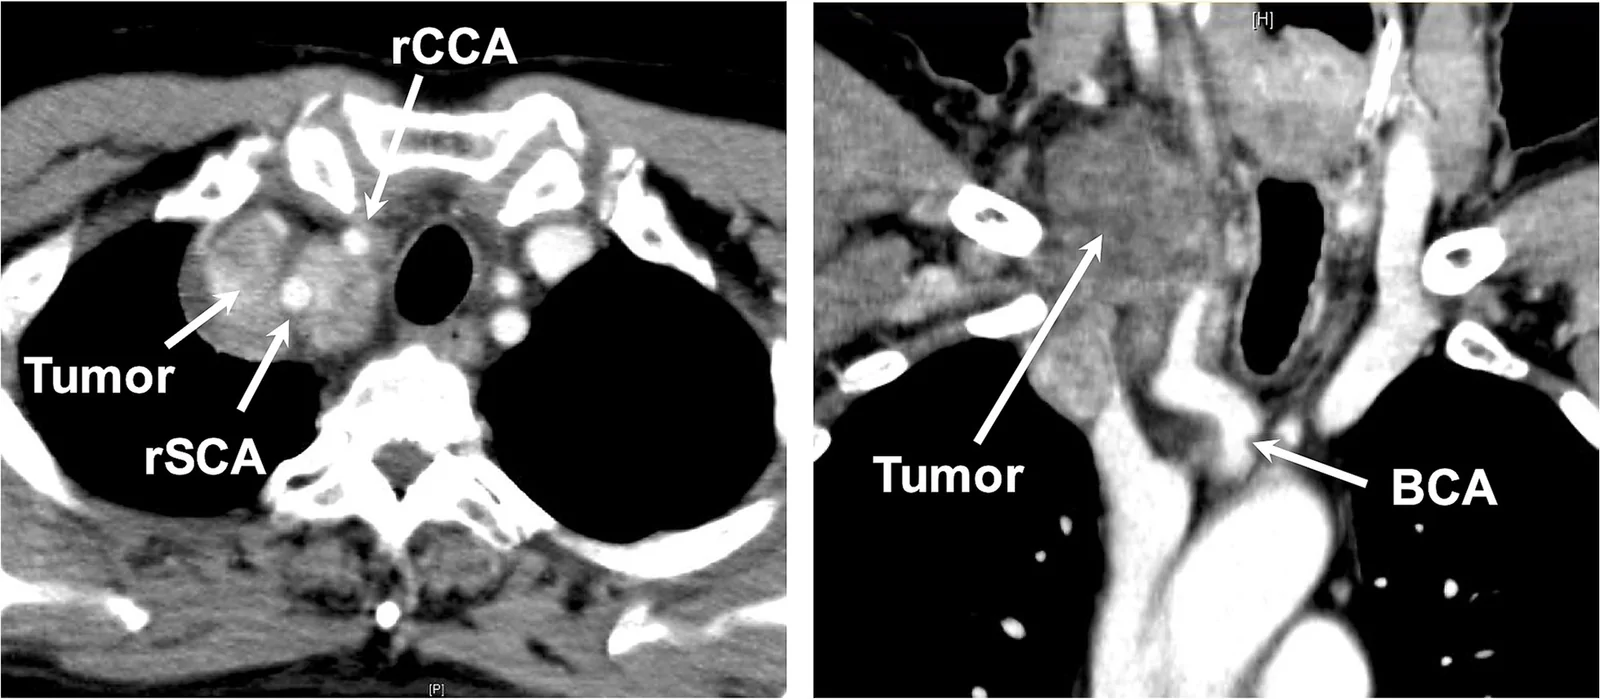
Contrast-enhanced CT before our first operation. The tumor surrounded the right subclavian artery and right common carotid artery. BCA, brachiocephalic artery; rCCA, right common carotid artery; rSCA, right subclavian artery.
